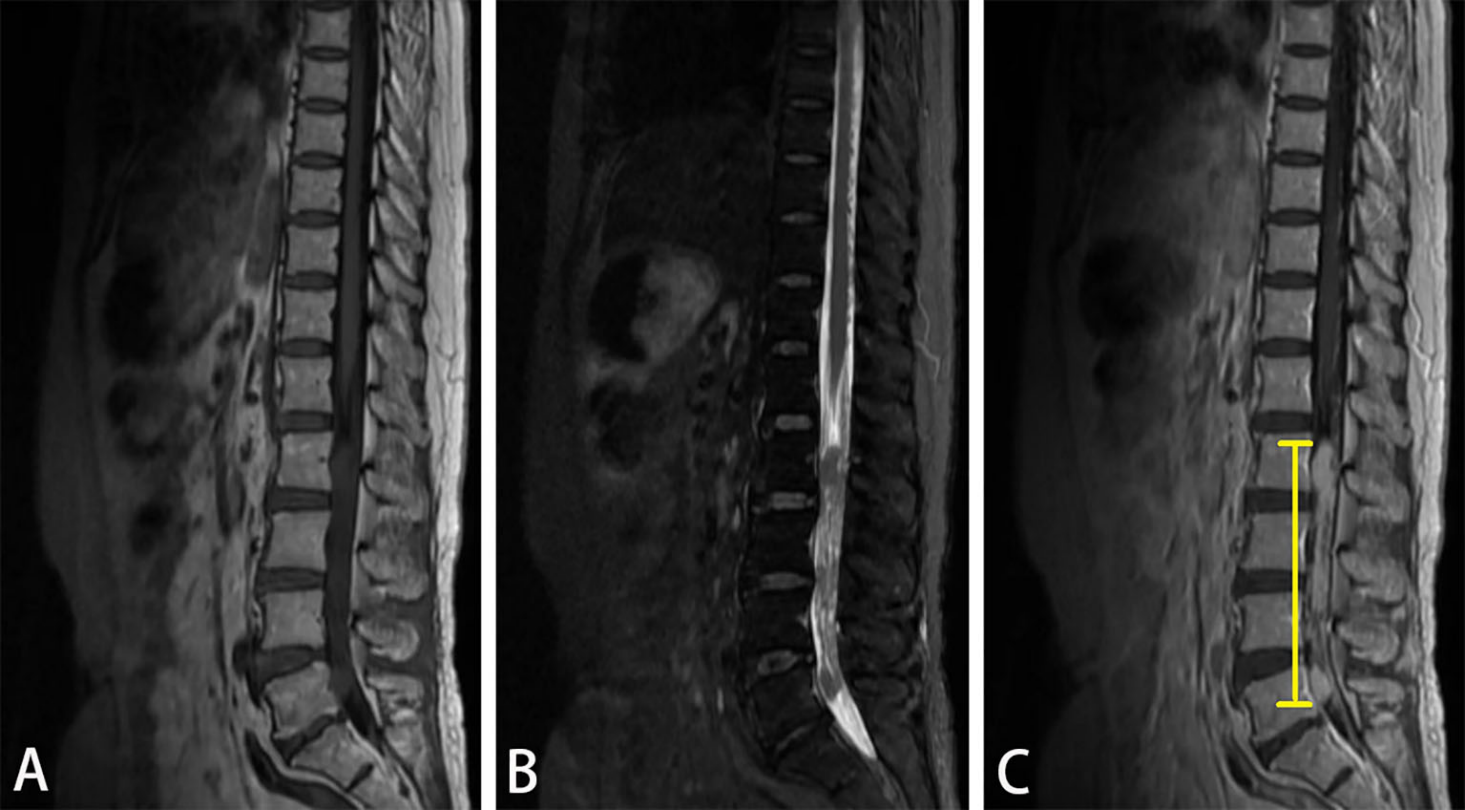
Magnetic resonance imaging (MRI) of the patient’s thoracic and lumbar vertebrae on the admission. Lumbar spine magnetic resonance imaging revealed a space-occupying lesion at the level of the second through fifth lumbar vertebrae. T1-weighted fluid-attenuated inversion recovery MRI (A) showed low signal intensity and inhomogeneously low signal intensity on T2-weighted MRI (B). The lesion (indicated by yellow lines) showed a remarkable enhancement on contrast-enhanced T1-weighted MRI (C).
